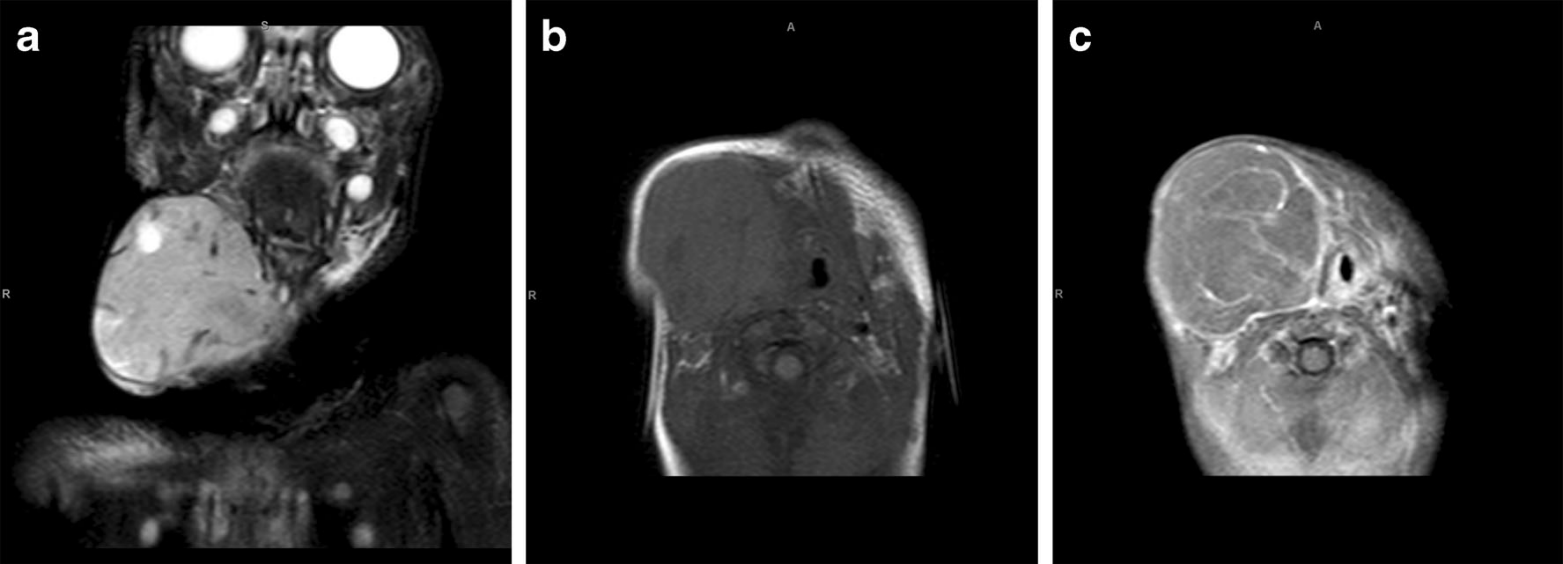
Fetiform teratoma. Coronal T2-W (a) and axial T1 W (b) MRI performed at 1 month of age shows a well-defined solid mass medial to the ramus of the mandible on the right. There are small hypointense foci on the T2-W image (a), and the mass enhances poorly post-contrast (c)
